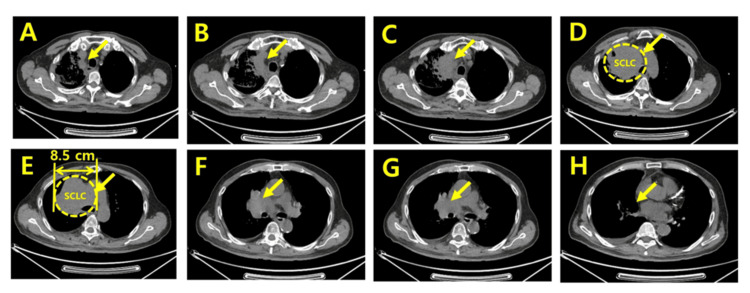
Baseline axial CT images captured immediately after diagnosis (2025-06-05, Slices A–H) A series of eight axial CT slices (A–H) from the thoracic inlet to the cardiac level obtained at initial diagnosis. Slices A–D reveal a large, irregular anterior mediastinal and right paratracheal mass with heterogeneous internal density and compression of adjacent mediastinal structures. Slices E–H show inferior extension of the mass with continued displacement of the mediastinum and partial effacement of the pericardial border. These images represent the full cranio-caudal extent of the primary tumor burden before systemic therapy. Arrows indicate small cell lung cancer sites.
